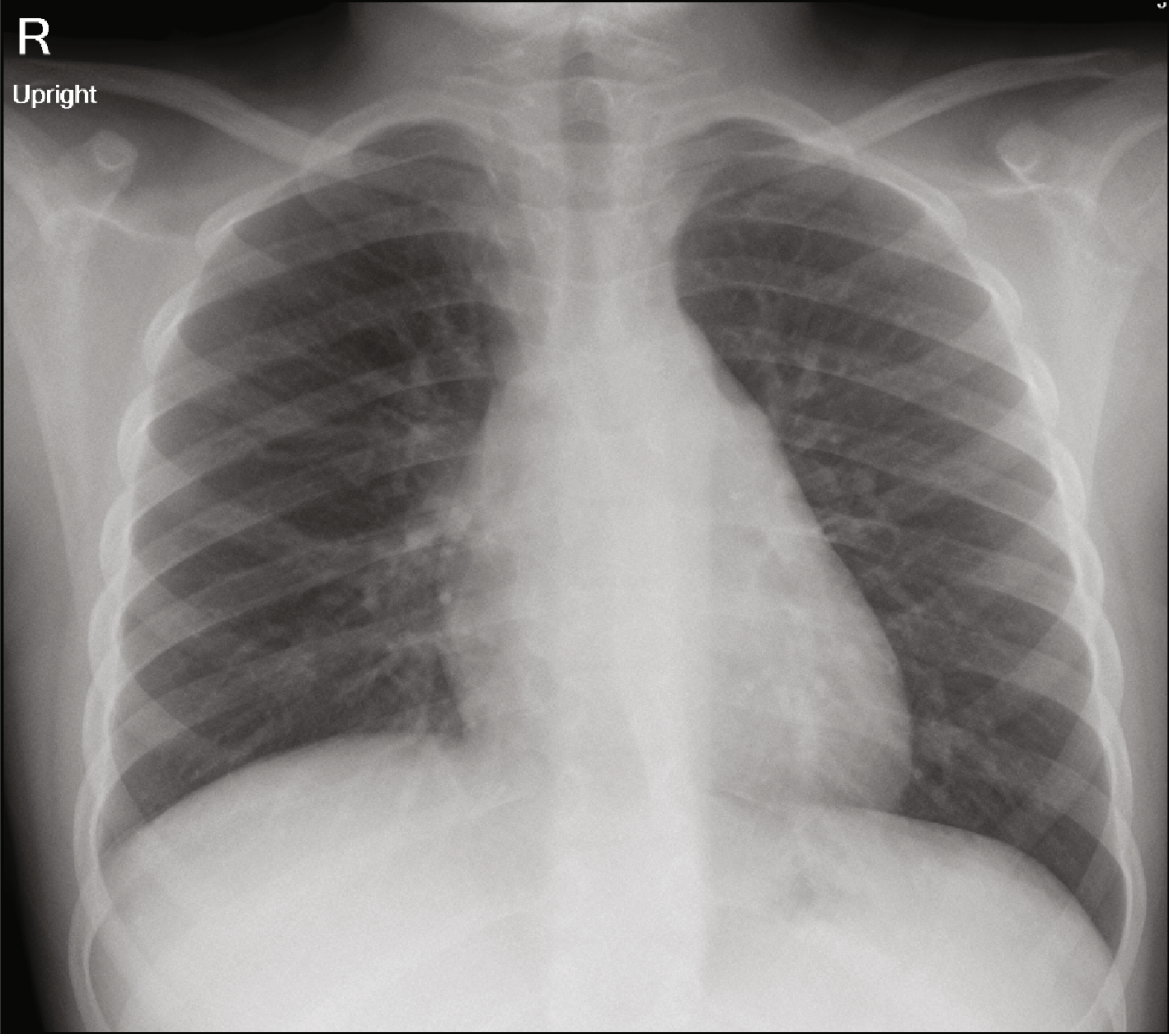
PA view of chest X-ray at the 4-month follow-up visit: Both sides of the lungs were well expanded and clear, with no residual changes noted in the middle lobe. There was no pleural effusion, only trace thickening at the right basal pleura.
